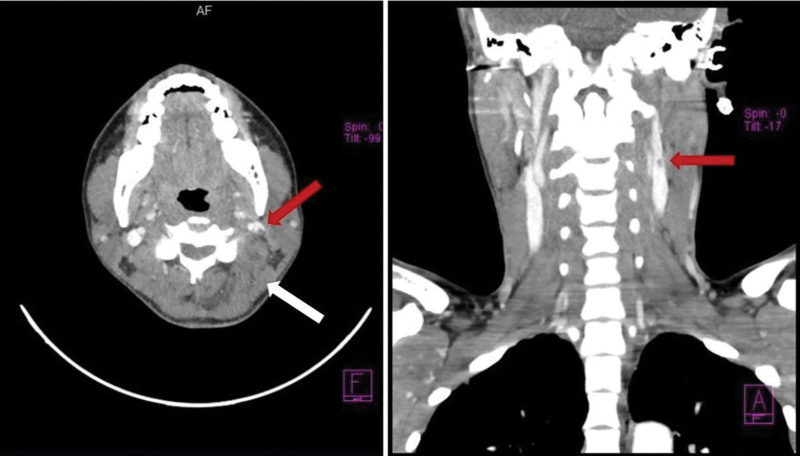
On admission, a contrast neck CT scan showed increased volume and inflammation of subcutaneous cellular tissue (white arrow) and hypodensity in the jugular vein (red arrows).
